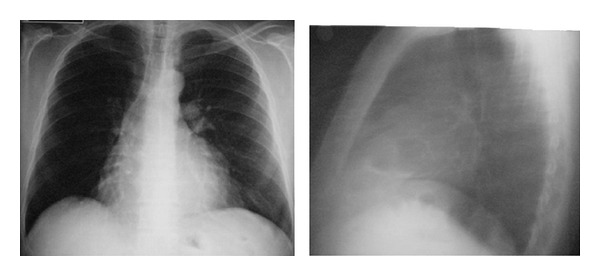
Posteroanterior and lateral chest radiography showing a shadow which superimposed on the shadow of his heart.
